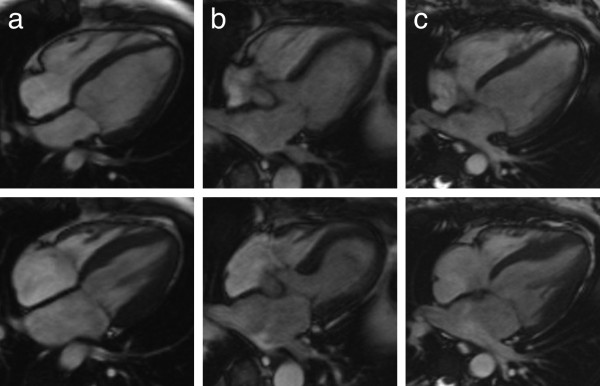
Native cardiac magnetic resonance imaging cine sequences. The row above shows the end diastolic, the row below the end systolic state of the cardiac cycle. Column a shows a patient with normal left ventricular function and a symmetric contractility of the myocardium throughout the left ventricle, b shows a patient with takotsubo cardiomyopathy with normal contractility of the basal myocardium and impaired apical contractility with ballooning, c is the patient of this case report with reverse (inverted) takotsubo cardiomyopathy with normal contractility of the apical myocardium and impaired contractility of the basal parts.
